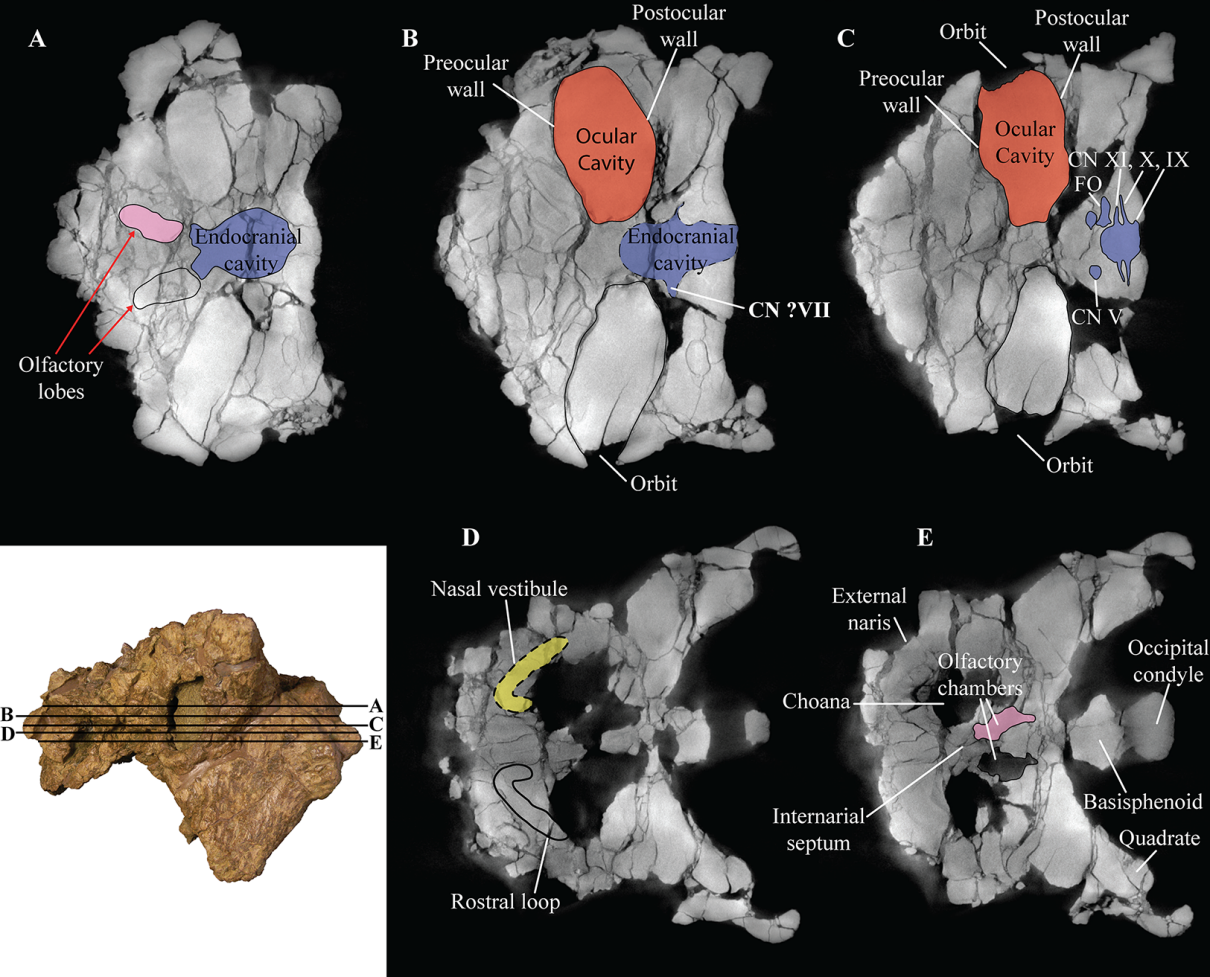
Computed tomography (CT) scans of *Akainacephalus johnsoni* (UMNH VP 20202). Transverse plane CT visualization of the skull of *Akainacephalus johnsoni* revealing important anatomical features, otherwise obscured by bone and/or matrix. Anatomical features in skull of *A. johnsoni* with horizontal lines indicating localities for the CT slices; (A), oblong-shaped olfactory lobes and outline of the endocranial cavity which provides space for the brain, including its olfactory lobes; (B), and (C), ocular cavities and their surrounding pre- and postocular walls. Presence of cranial nerves CN V, ?VII, IX, X, XI and the fenestra ovalis (FO) along the lateral surfaces of the endocranial cavity; (D), anatomical outline of a small portion of the complex nasal air passage system showing the rostral loop of the nasal vestibule; (E) outlines of the olfactory chambers which house the olfactory lobes. Images not to scale.
